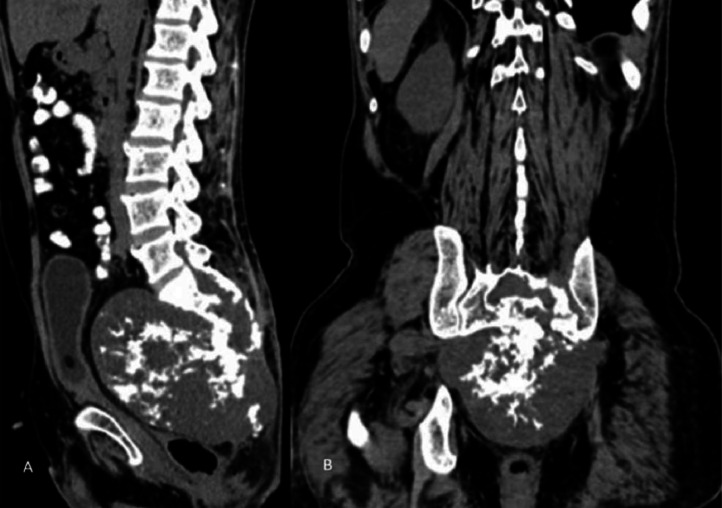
CT sagittal (A) and coronal (B) sections showing large well circumscribed expansile soft tissue density mass lesion with intralesional calcifications noted in the pelvis in the region of lower sacrum and coccyx causing its destruction.
